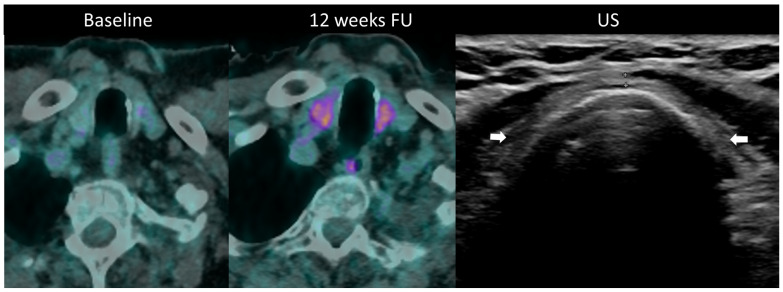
Immune-related thyroiditis in a patient receiving chemotherapy and combined anti-PD-1 + anti-CTLA-4 for metastatic lung cancer. At baseline 18F-FDG PET/CT, no thyroid uptake is seen. At week 16, a diffuse moderate metabolic activity of the thyroid gland appeared at PET/CT. The patient developed a grade 2 primary hyperthyroidism. After the resolution of the acute phase, the laboratory tests showed a marked primary hypothyroidism and the ultrasound depicted an atrophic and heterogeneously hypoechogenic thyroid gland (arrows) consistent with a pre-existing chronic thyroiditis.
